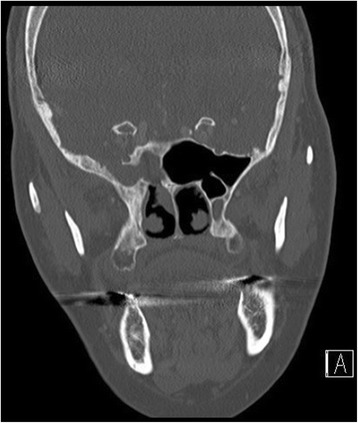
Coronal CT showing right lateral sphenoid defect and encephalocele in patient #5
